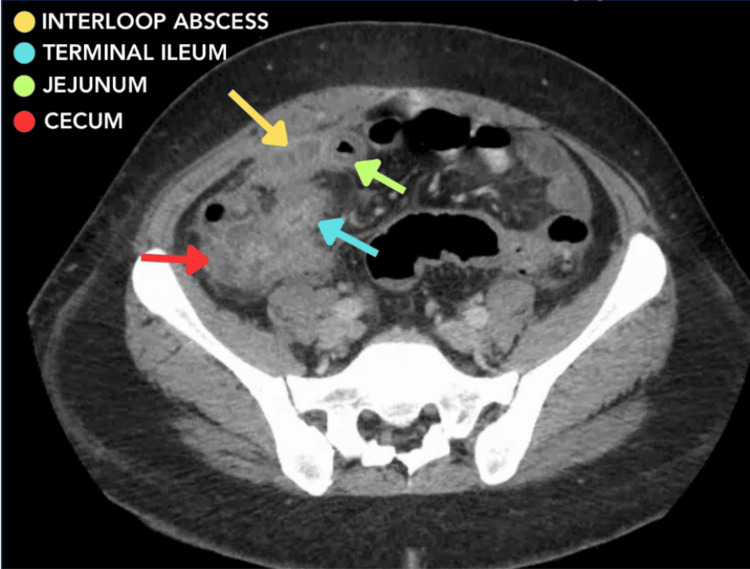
Axial view of patient’s presurgical CT of the abdomen and pelvis
